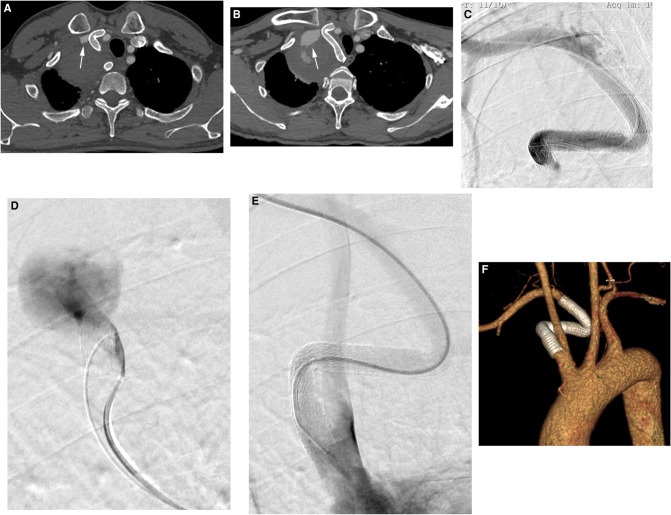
(A) CTA after 1 week indicated that the SAA was essentially occluded with a patent stent graft, and thrombus formation was observed in the aneurysm (white arrow). (B) At the 6-month follow-up, the aneurysm was not obviously shrinking, and CTA revealed that there was still an endoleak into the aneurysmal sac (white arrow). (C,D) Angiography revealed no contrast material leakage through the fabric of the inflexion of the stent graft, confirming a type Ia endoleak and the stent graft was caudally displaced. (E) Angiography showed successful exclusion of the aneurysm, with the absence of a type I endoleak. (F) At the 25-month follow-up, CTA revealed no evidence of an endoleak into the aneurysmal sac, and the stent graft was patent.
